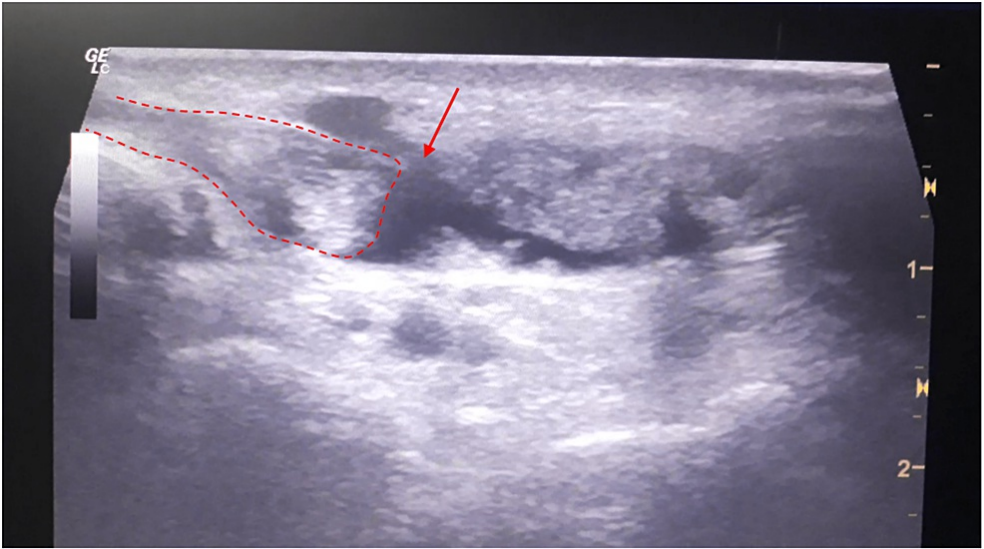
Ultrasound image showing discontinuous extensor pollicis longus in longitudinal scans (red dots) with hematoma (arrow)
